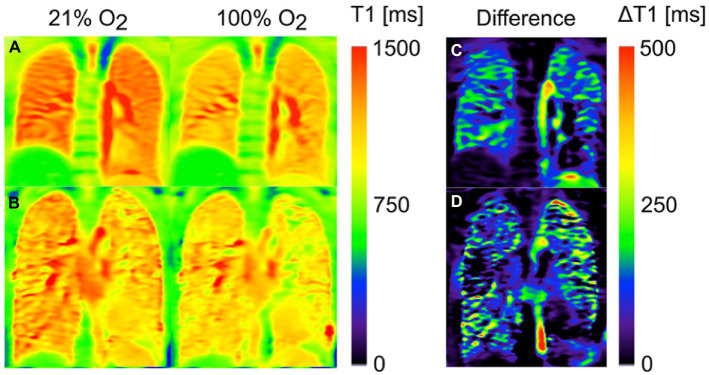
Oxygen-enhanced MRI T1 maps of a healthy volunteer (A) and a patient with cystic fibrosis (CF) (B) after inhalation of 21 and 100% oxygen. The third column shows the corresponding difference maps: While a homogenous reduction of T1(100% O2) in comparison with T1(21% O2) is found for the healthy volunteer (C), some regions of the CF patient (D) show no or only small changes. Assuming that this effect can be mainly ascribed to reduced regional oxygen supply, the difference map can be interpreted as a surrogate for ventilation. Adapted with permission from (234).
